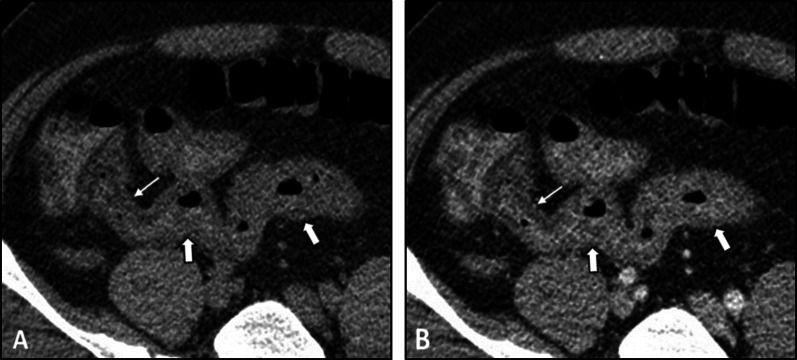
Intestinal ischemia in a 37-year-old male with a history of known Henoch–Schönlein purpura (HSP) presenting with abdominal pain and bloody diarrhea. The patient underwent pre (A)- and post (B)-IV contrast CT scans using positive dilute oral contrast. Segmental mural thickening (thick white arrows) is seen in the terminal ileum with a focal area of mural hypoenhancement (thin white arrows) proximal to the ileocecal valve. Ileocolonoscopy confirmed mucosal ischemic changes, and signs/symptoms were improved following treatment by corticosteroid
